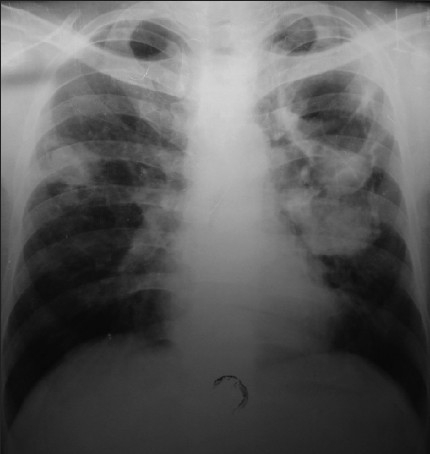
Chest radiograph after two year of antitubercular treatment showing increased cavitation along with fungus ball and progression of disease to right side
